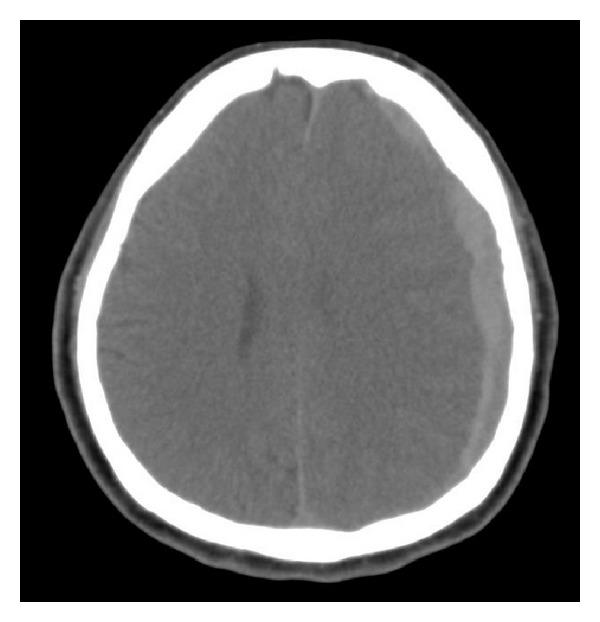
Brain CT (noncontrast), axial view. Subacute subdural hemorrhage was found after head trauma from a fall.
